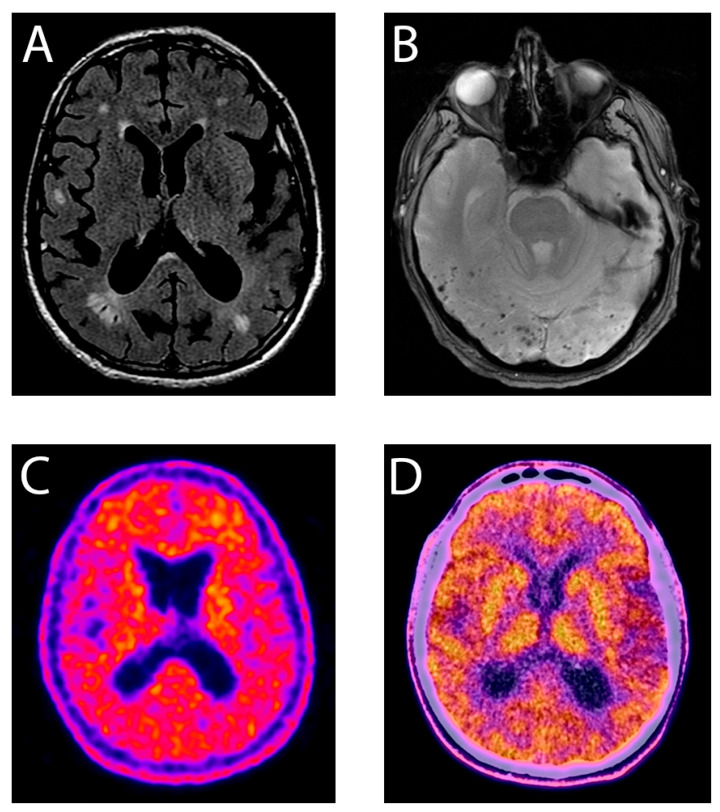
FLAIR (A) and echo-gradient (B) MRI sections showing hyperintense periventricular and subcortical lesions and multiple lobar microhemorrhages compatible with CAA, respectively. PiB PET and 18-FDG scan demonstrating generalized cortical amyloid deposition (C) and subtle decrease in metabolism in the insular area (D).
